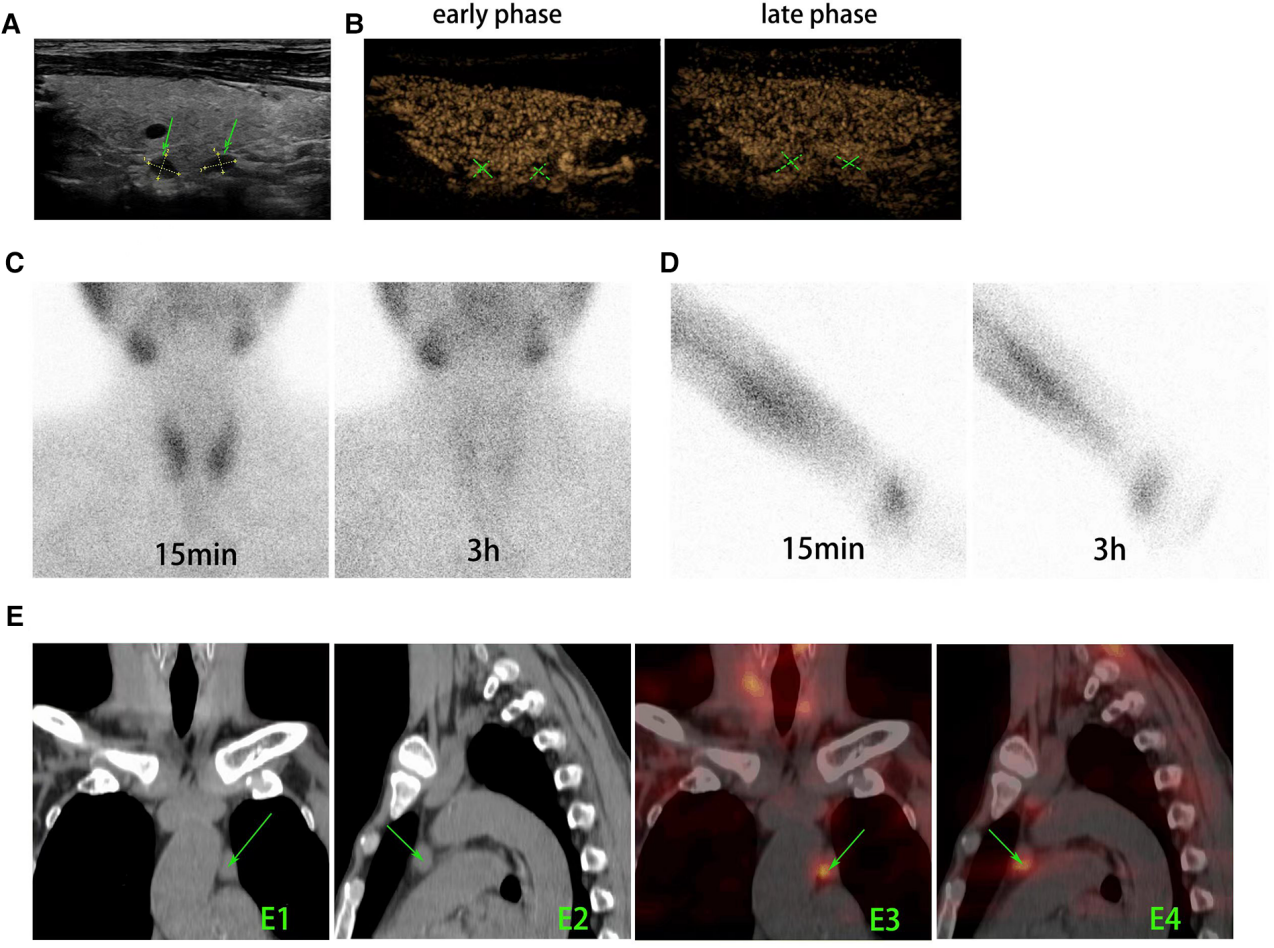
Preoperative imaging modalities were used to find and locate the parathyroid tissues. (A) US revealed two hypoechoic lesions with volumes of 0.55 cm × 0.45 cm and 0.55 cm × 0.35 cm (arrow) located at the dorsal area of right lobe of thyroid gland. (B) In CEUS, both lesions appeared as characteristics of parathyroid tissues (fast in the early phase, slow out in the late phase, and higher enhancement with a long enhancement time). (C) ^99m^Tc-MIBI planar early (15 min) and delayed (3 h) static images showed that there is no tissue with focal tracer accumulation presented at the primary surgery site of neck. (D) ^99m^Tc-MIBI planar early (15 min) and delayed (3 h) static images showed that there is no focal tracer accumulation at the autograft site. (E) The axial CT (E1 and E2) and SPECT/CT (E3 and E4) images showed soft-tissue masses with marked MIBI uptake in the left upper mediastinum (arrows). US, ultrasound; CEUS, contrast-enhanced ultrasound; ^99m^Tc-MIBI, ^99m^Tc-sestamibi.
